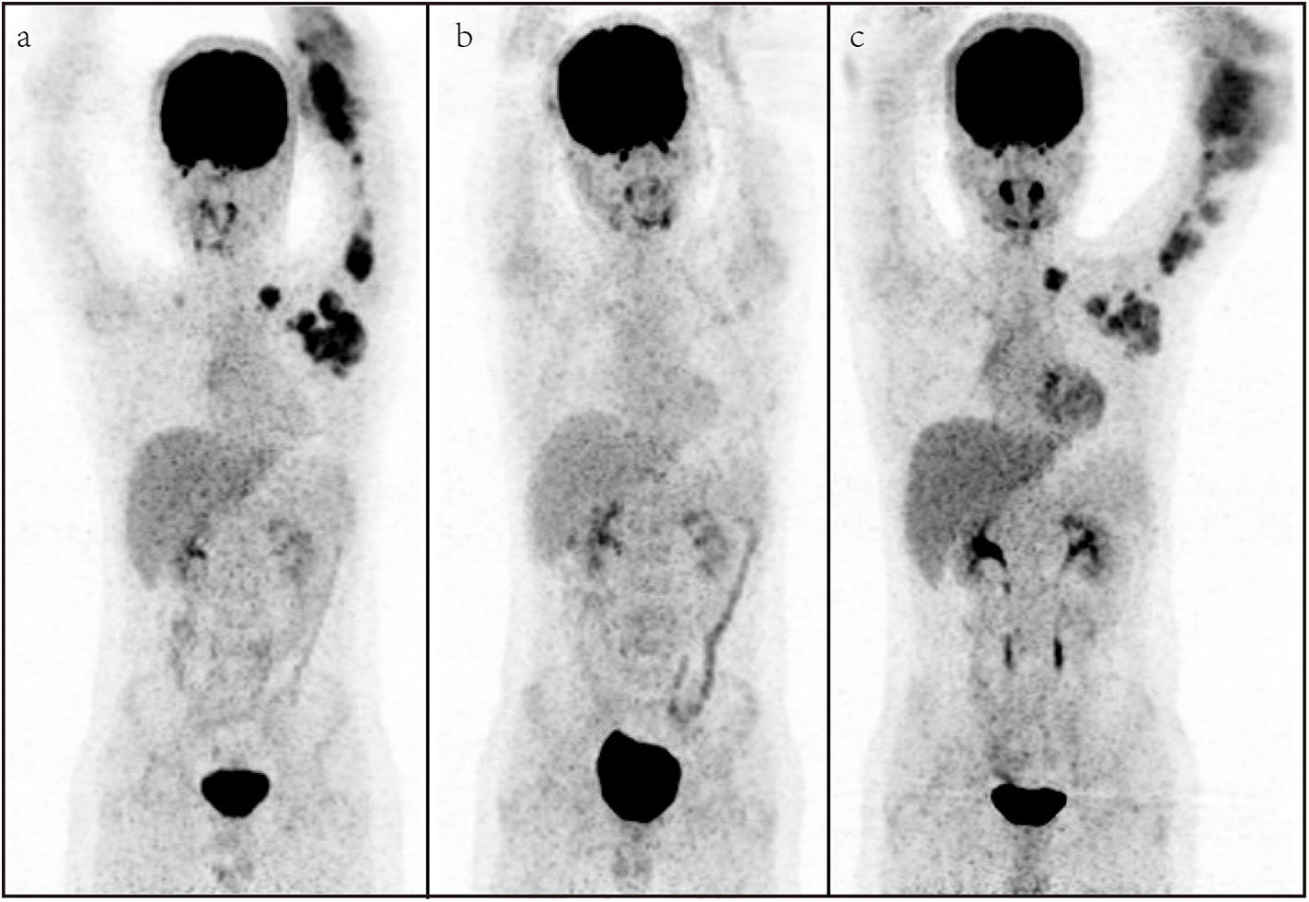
A representative case of a 13-year-old male with rhabdomyosarcoma. Baseline maximum intensity projection (MIP, a), showing hypermetabolic disease in laterocervical, mediastinal, axillary, elbow, and hilar lymph nodes and humerus [maximum standardized uptake volume (SUV_max_): 9.2, SUV_mean_: 4.3, SUV_peak_: 6.9, metabolic tumor volume (MTV): 264.6 cm^3^, total lesion glycolysis (TLG): 1,202.5]. PET/CT (b) after therapy showing a partial metabolic response with fluoro-deoxyglucose (FDG) uptake lymph nodes in the axillary. PET/CT (c) for follow-up showing metabolic progression of disease with appearance of new lesions.
